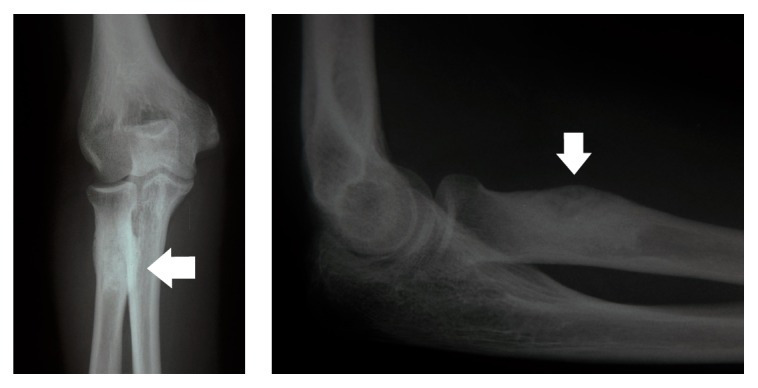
Radiograph images of the patient's elbow upon initial examination. Periostitis' signs and an image of “onion leaves” along with bone sclerosis at the bicipital tuberosity of the radius.
